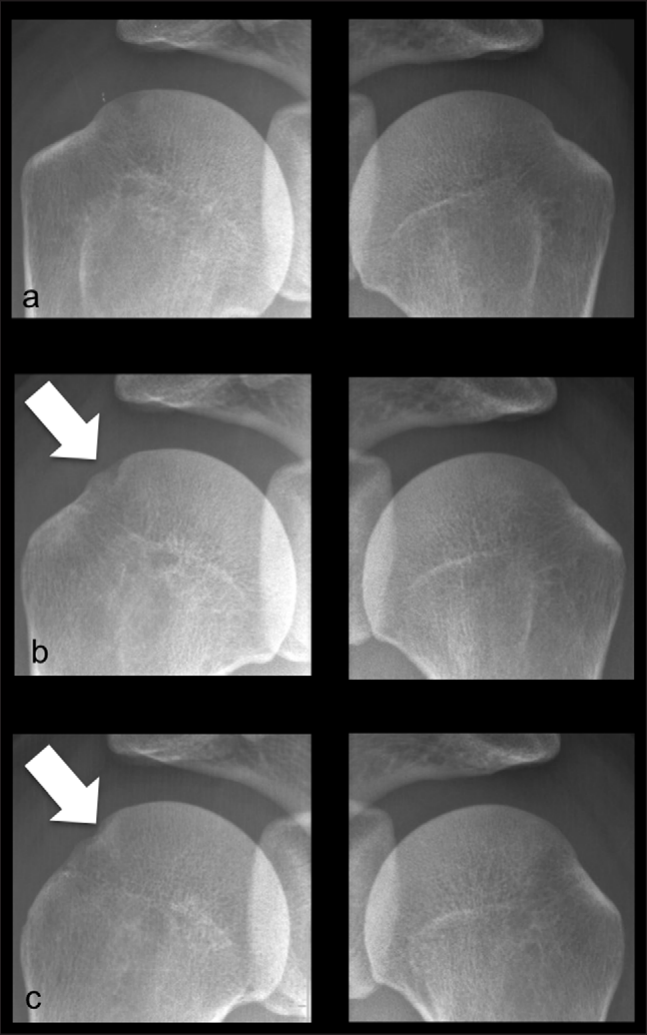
Bilateral frontal shoulder radiographs obtained with varying degrees of rotation of the humeral head, from neutral (a) to internal rotation (c), without any modification to the beam angulation. The Hill-Sachs lesion (arrow), is not visible in neutral rotation. The pathological nature of the cortical irregularity of the postero-superior aspect of the humeral head can be affirmed in view of the normal aspect of contralateral asymptomatic left side.
